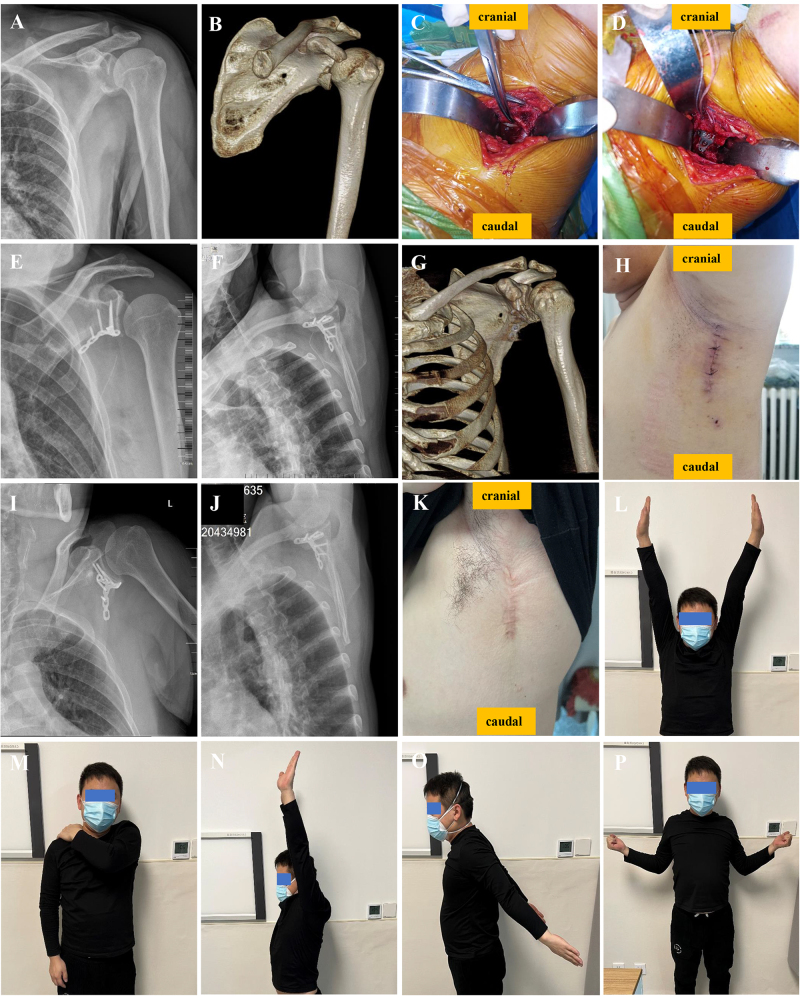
The technique and outcomes associated with the T-W approach for an Ideberg Ia fracture. (A and B) Preoperative radiography and 3D-CT assessments. (C and D) Intraoperative photograph showing the fracture exposed and fixed with one plate. (E–H) Postoperative radiograph, 3D-CT image, and incision. (I–P) Radiograph, incision, and shoulder joint activity 14 months postoperatively.
